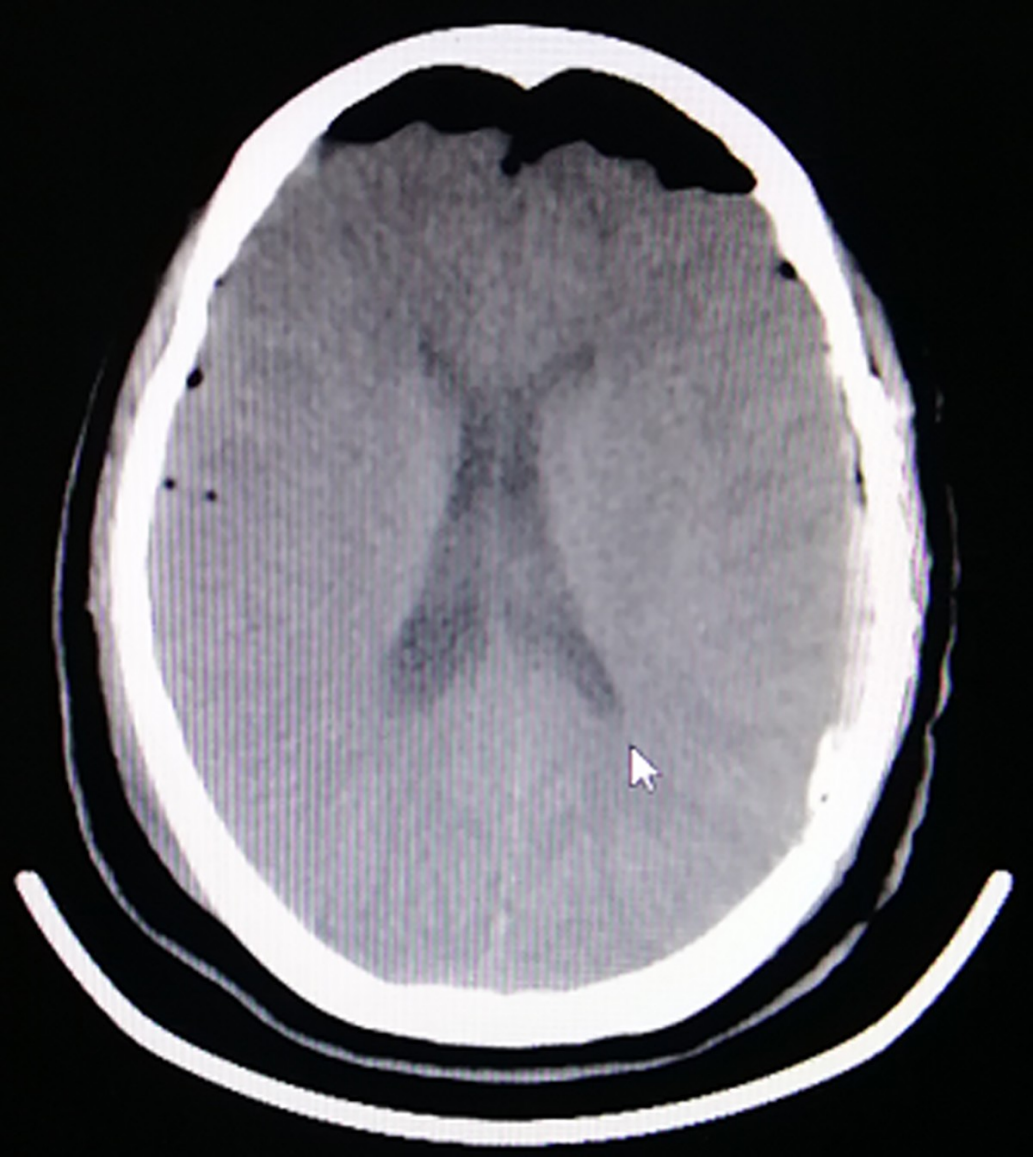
A postoperative computed tomography scan (axial view) showing intracranial air compressing the frontal lobes with widening of the interhemispheric space between the frontal poles, i.e., the Mt. Fuji sign.
